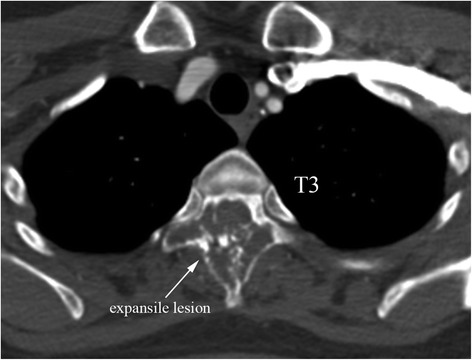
Preoperative axial CT scans showing an expansile lesion of osteolysis at the posterior elements of T3 vertebra, involving spinous process, lamina, right-side transverse process and pedicle (*white arrow*)
